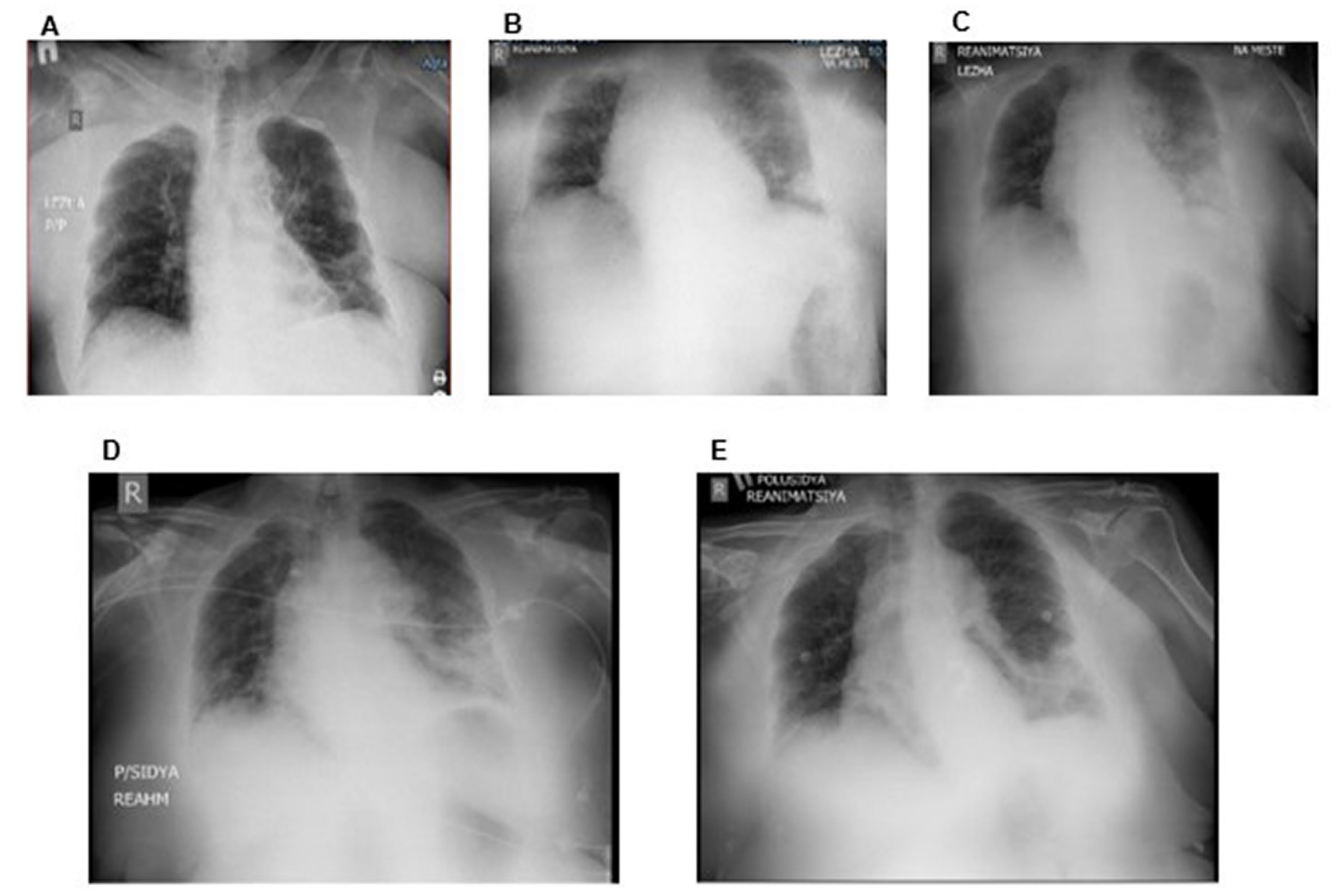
Dynamics of chest X-ray after adding a continuous infusion of meropenem: CXR on the 1st day (A), CXR on the 2nd day (B), CXR on the 3rd day (C), CXR on the 5th day (D), and CXR after 6 days of continuous infusion of meropenem (11th day of hospitalization) (E).
